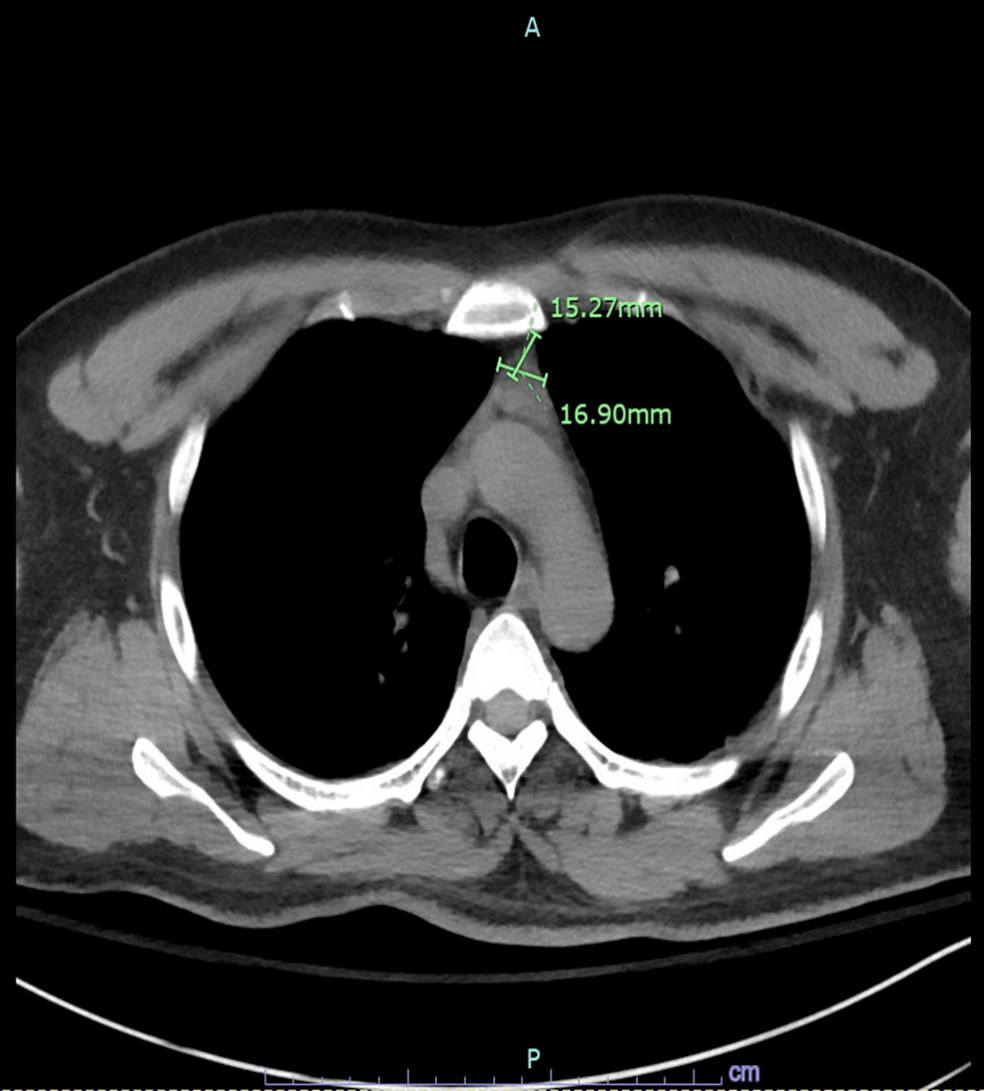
Thymic mass Computed tomography of the chest showing anterior mediastinal mass.
